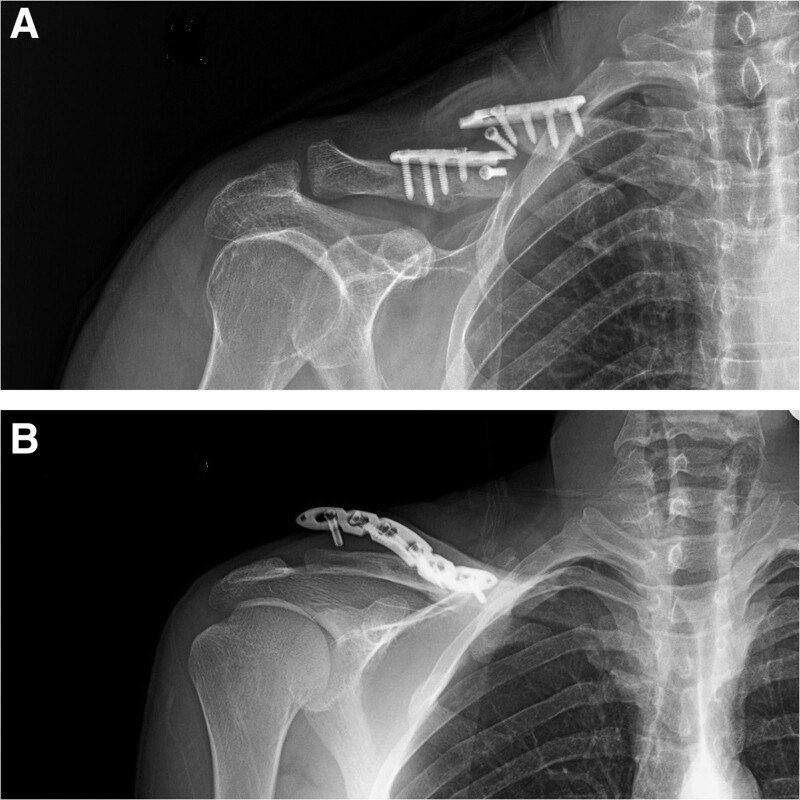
(A) X-ray of implant failure (breakage) at 3rd month postoperatively, 58 years old, male. (B) X-ray of implant failure at 2nd month postoperatively, 63 years old, male.
